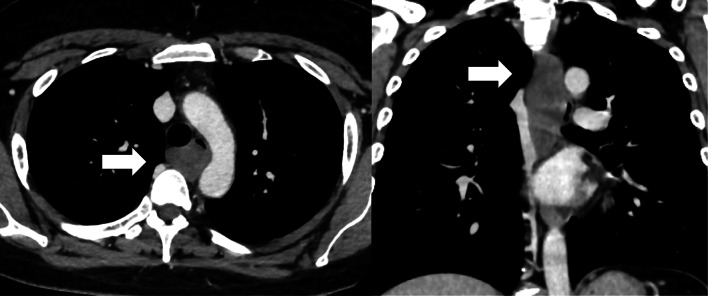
Enhanced computed tomography images
